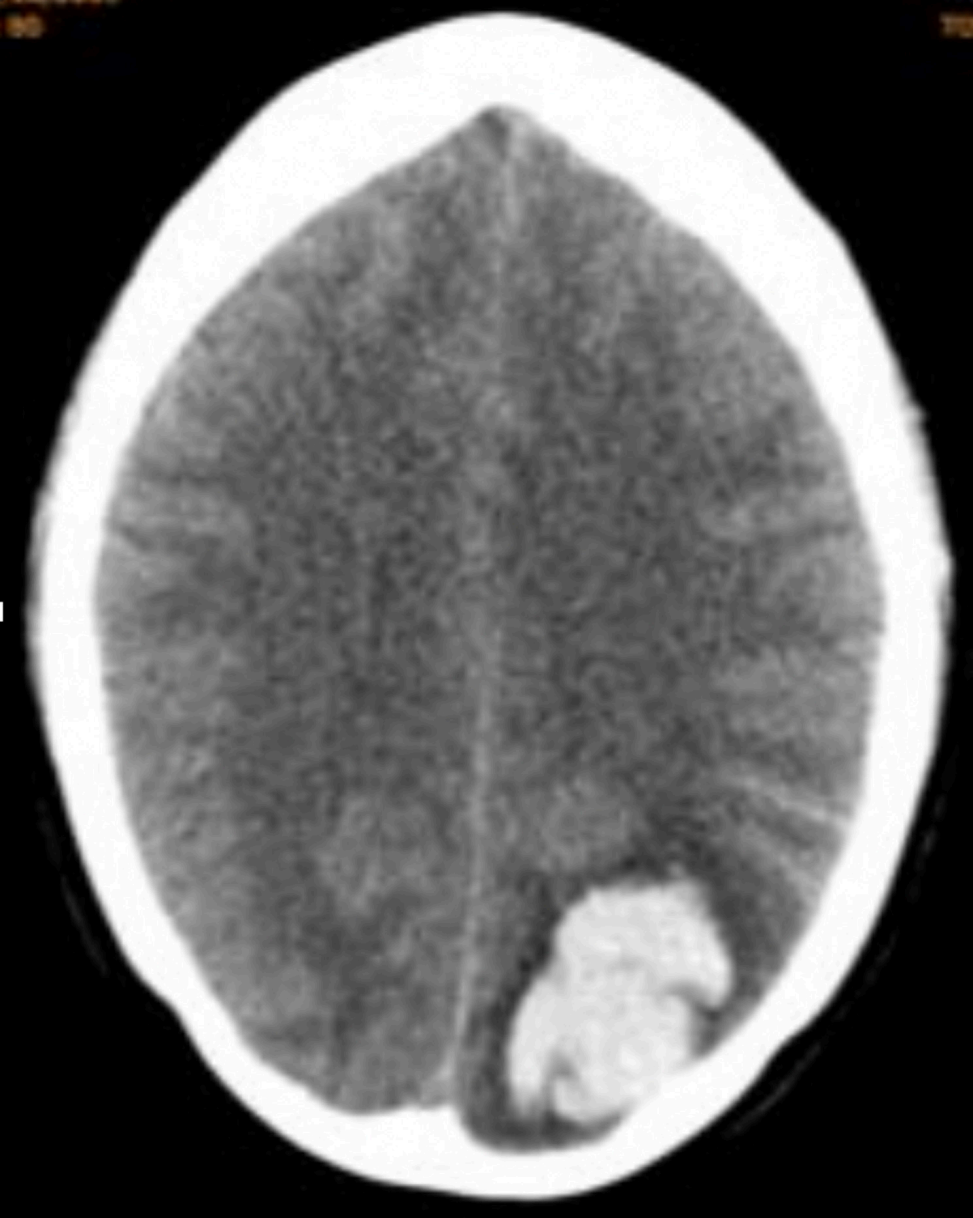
Head CT showing acute intraparenchymal haematoma within the left parieto-occipital lobe
